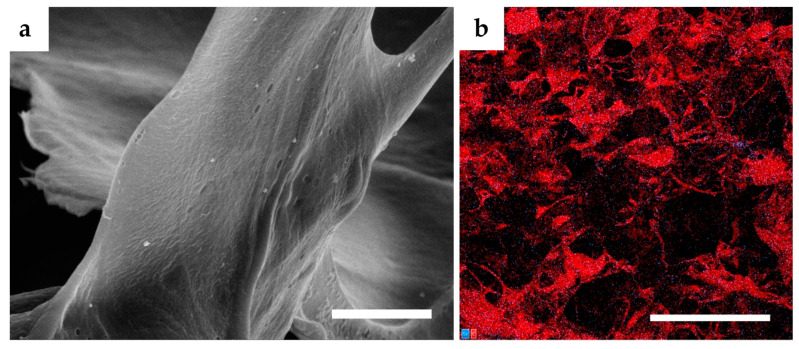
(a) SEM image of the C/SeCH scaffold acquired at 25,000× magnification with a scale of 5 µm; (b) energy-dispersive X-ray analysis image of the C/SeCH scaffold acquired at 175× magnification with a scale of 500 µm. The red color represents carbon elements and the blue color represents selenium elements.
